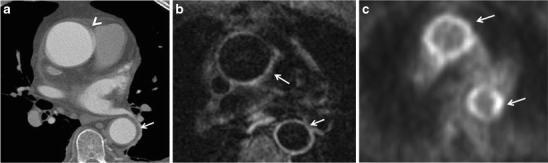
a–c A 59-year-old woman with low-grade fever, malaise and high white blood cell count. Axial (a) CTA image demonstrates normal thickness (*arrowhead*) of the ascending aortic wall with minimal thickening of the descending aortic wall (*arrow*). Axial contrast-enhanced MR image (b) shows contrast in the ascending and descending aortic wall corresponding to vivid 18-FDG uptake on PET-CT image (c)Hybrid imaging with 18F-FDG PET and either CTA or MRA allows more precise anatomic localisation of disease activity with increased uptake of 18F-FDG thought to be a surrogate marker of increased activity of inflammatory cells. The presence of wall thickening, arterial stenosis, luminal thrombus and aneurysm cannot be assessed by PET alone; CTA and MRA are complementary to PET for complete evaluation of the patient with aortitis [24].
